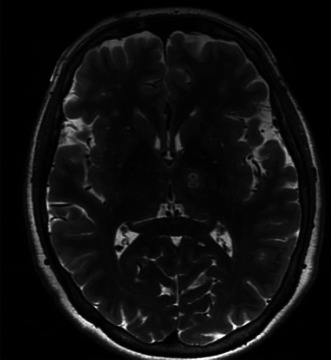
FIGURE 2 Axial T2 MRI sequence showing dual lesion at 1‐month follow‐up.
